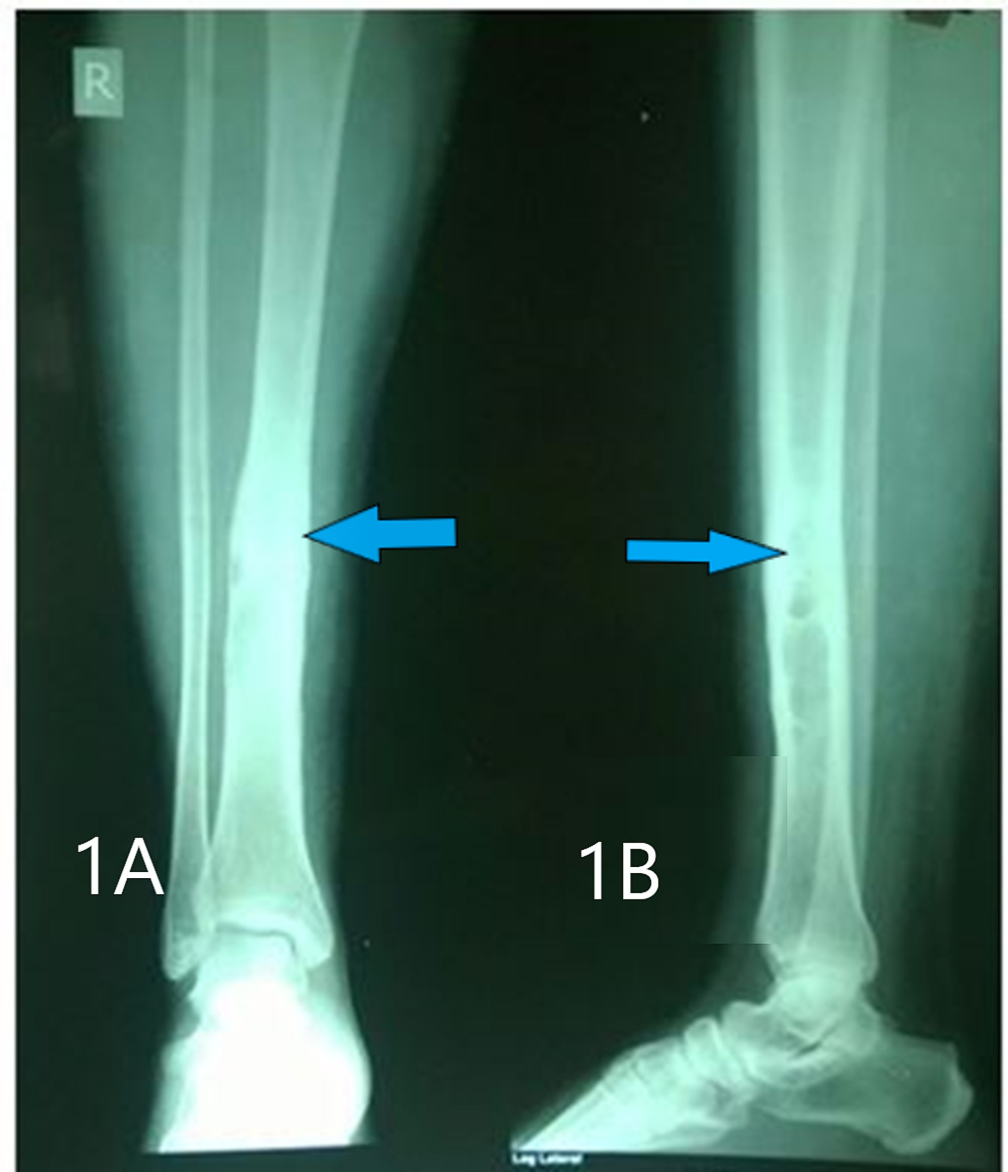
Pre-operative X-ray showing changes suggestive of osteomyelitis. Figures 1A, 1B show the anteroposterior and lateral views of the right tibia showing periosteal thickening, loss of corticomedullary differentiation, and lytic changes.
